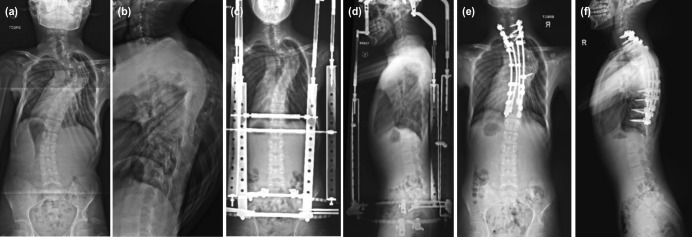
Plain radiographs (posteroanterior and lateral view) of Case 3: (a, b) before operation, (c, d) while on halo-pelvic traction, and (e, f) after single-stage posterior spinal fusion.
